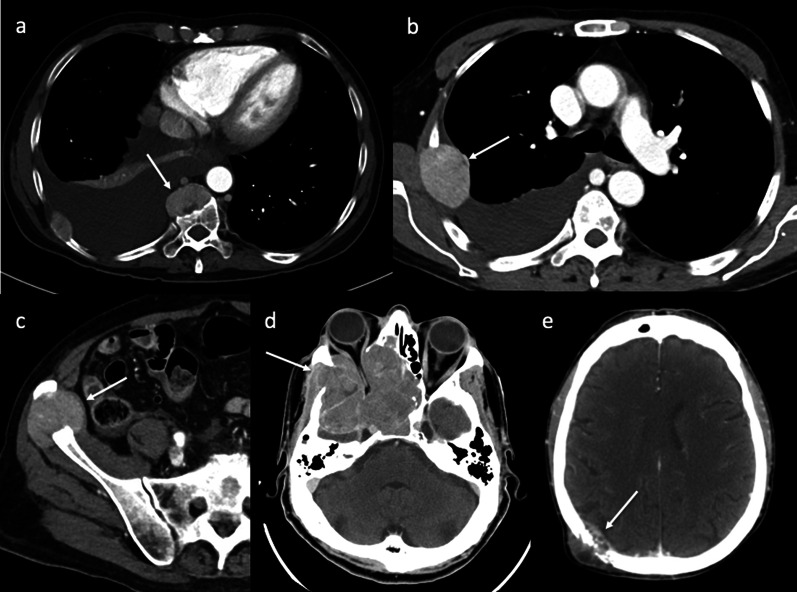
Bone recurrence. Axial contrast-enhanced CT images on the arterial phase (a–e) show bone recurrence of HCC (arrows) in different patients. Bone metastases from HCC are typically lytic (bone destruction), expansive, and characterized by soft tissue mass with typical hyperenhancement on the arterial phase. The sites of recurrence are vertebrae (a), ribs (b), right iliac bone (c), and skull (d, e). In patients with recurrent HCC in the right side of the anterior and middle cranial fossae (d), the lesion causes bone destruction with invasion of the orbit and ethmoid
